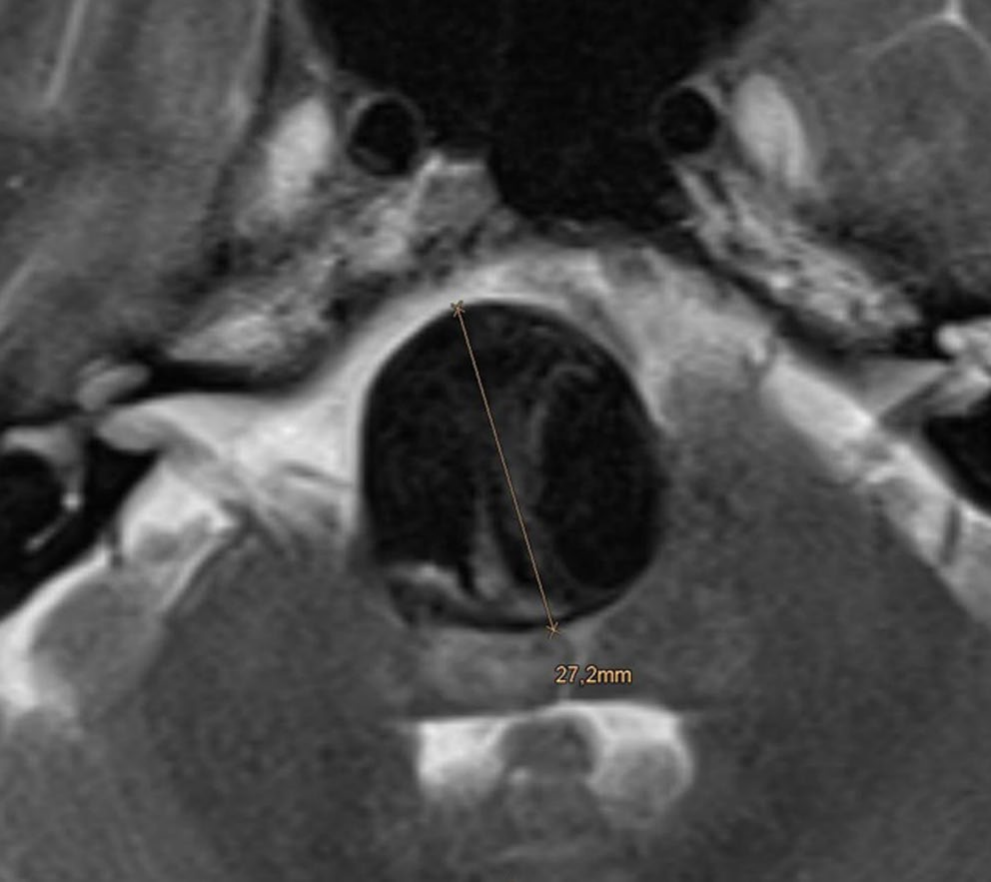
T2-weighted axial magnetic resonance imaging (MRI) of a wide necked partially thrombosed proximal basilar artery aneurysm, prior to treatment
